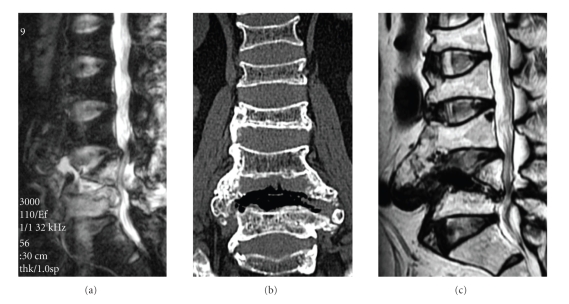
Imaging studies for Case 1. (a) Preoperative lumbar spine MRI showing sagittal T2-weighted image. Destruction of the L4 vertebral body and an abscess are evident. (b) Postoperative lumbar spine MRI showing sagittal T2-weighted image. Normalization of signal intensity in vertebral bodies and disappearance of abscess are observed. (c) Postoperative CT showing callus formation bridging the vertebral bodies around the L4/5 intervertebral region.
